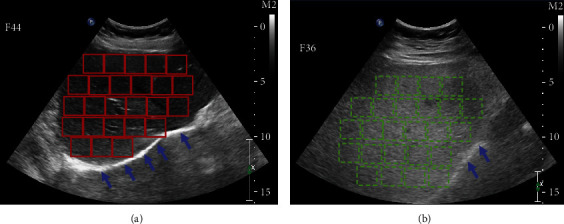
Example of generating image patches through the annotations of the ultrasonic liver images. (a) An ultrasonic image of normal liver. (b) An ultrasonic image of severe fatty liver. In the normal liver, the echo at the rear of the liver is obvious (marked by five blue arrows in (a)). When a large amount of fat particles is contained in the liver, the liver ultrasonic image turns white and the echo attenuation is serious (marked by two blue arrows in (b)).
